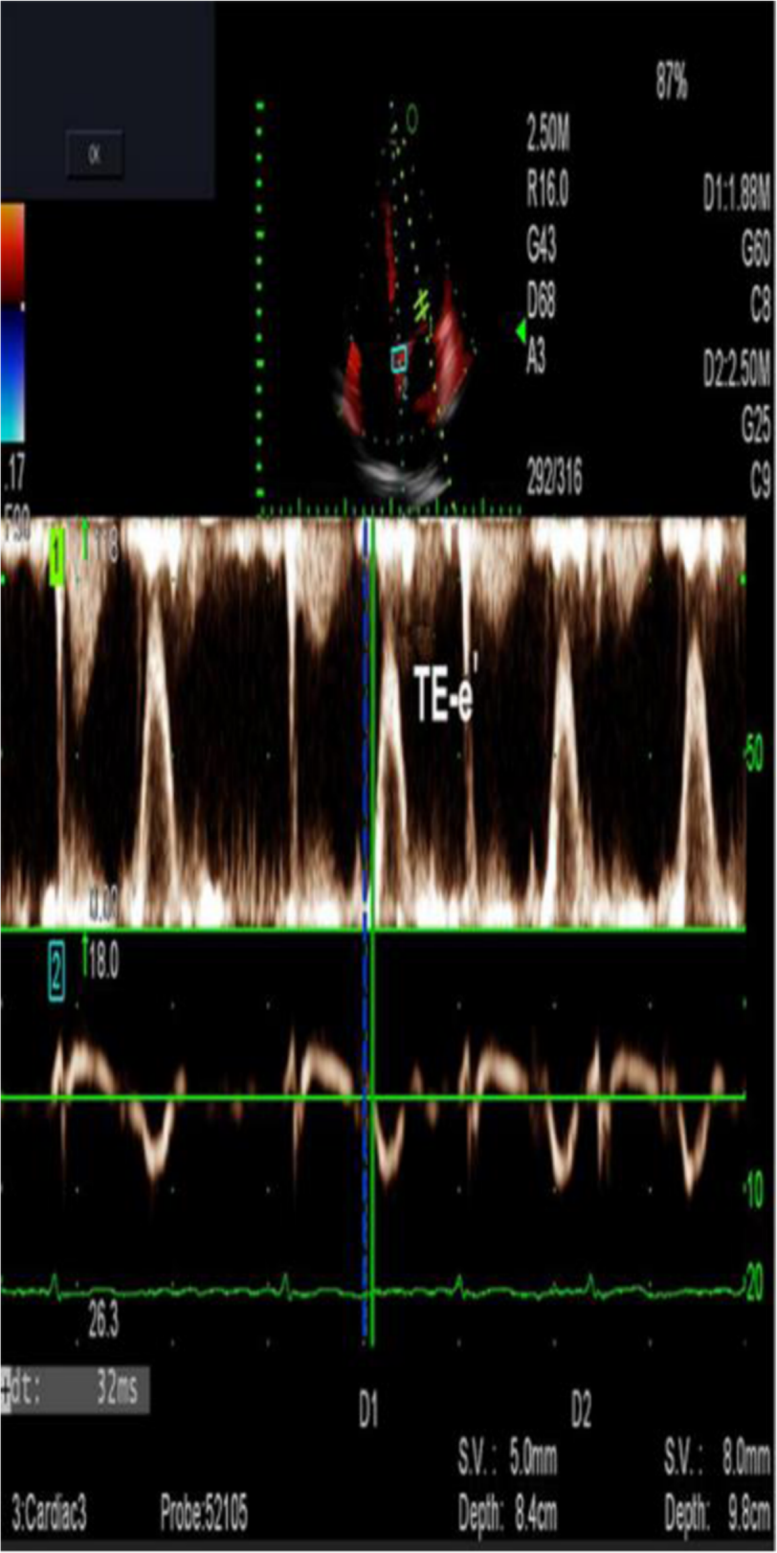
Measurement of TE-e’ using a dual Doppler system. From simultaneous recordings of transmitral inflow and mitral annular velocity, the time interval was measured between the onset of E and of e’(TE-e’) for 10 consecutive beats
